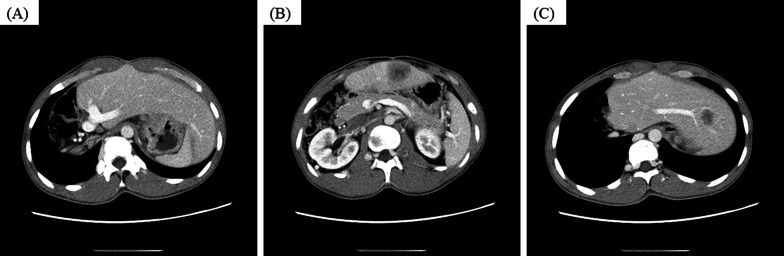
A Contrast-enhanced computed tomography revealing tumor invasion beyond the serosal layer. B, C Multiple metastatic lesions in the transplanted liver
